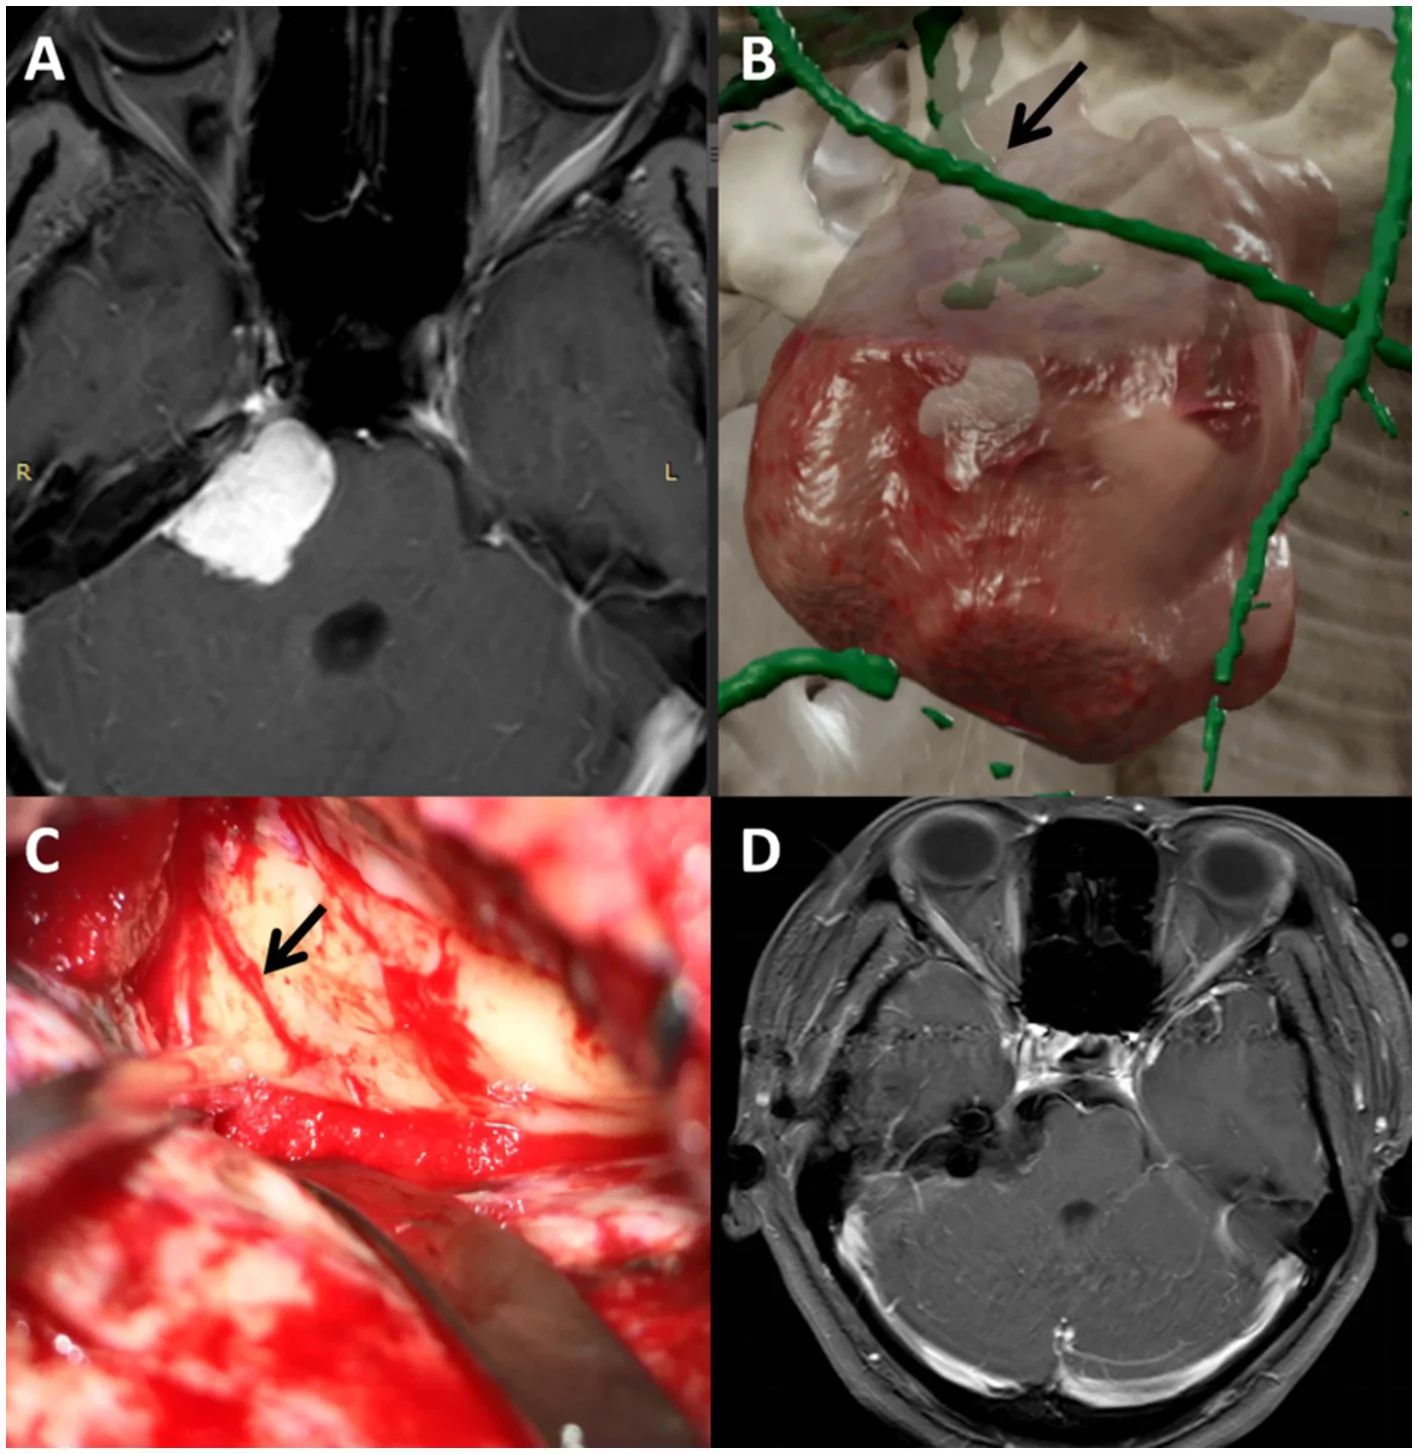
Petroclival meningioma with middle meningeal artery supply. (A) Preoperative contrast-enhanced MRI showing a right petroclival meningioma. (B,C) Preoperative MFI demonstrating tumor blood supply from the middle meningeal artery in the petrous bone region. The feeding artery is indicated by the black arrow. (D) Postoperative contrast-enhanced MRI showing Simpson grade I resection.
